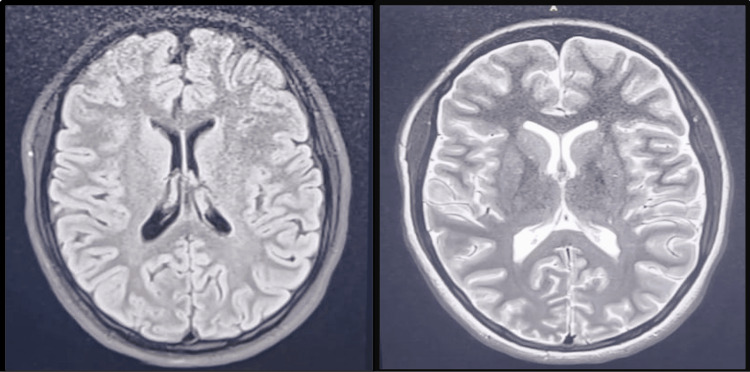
Axial T2 WI through the basal ganglia level appears unremarkable Axial T2 WI: axial T2-weighted images
